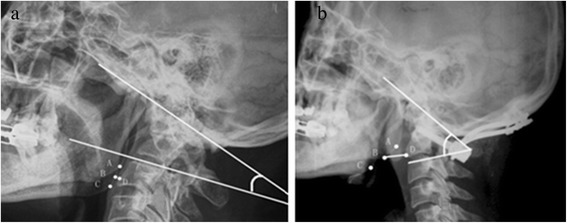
A preoperative and postoperative radiograph. (a) Preoperative radiograph of a patient with chronic atlantoaxial dislocation secondary to occipitalization showed a decreased MCD and OCA. After surgery (b), the MCD, OCA, and the curve of the cervical spine were all improved, although the MCD was somewhat longer than normal.
